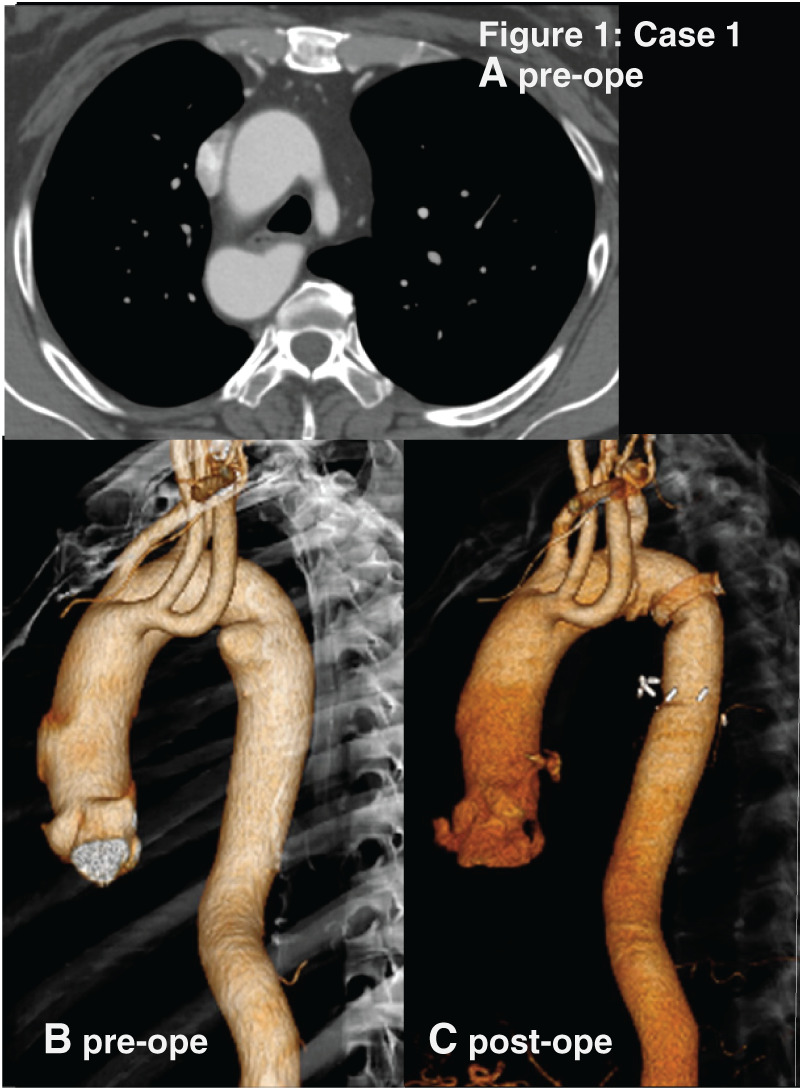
The figure 1 correspond to the images of Case 1 respectively. (A) Preoperative enhanced CT images, axial view. (B) Preoperative 3D-reconstructide CT. (C) Postoperative 3D-reconstructed CT. Case 1 are mirror-image RAAs. RAA, right-sided aortic arch
